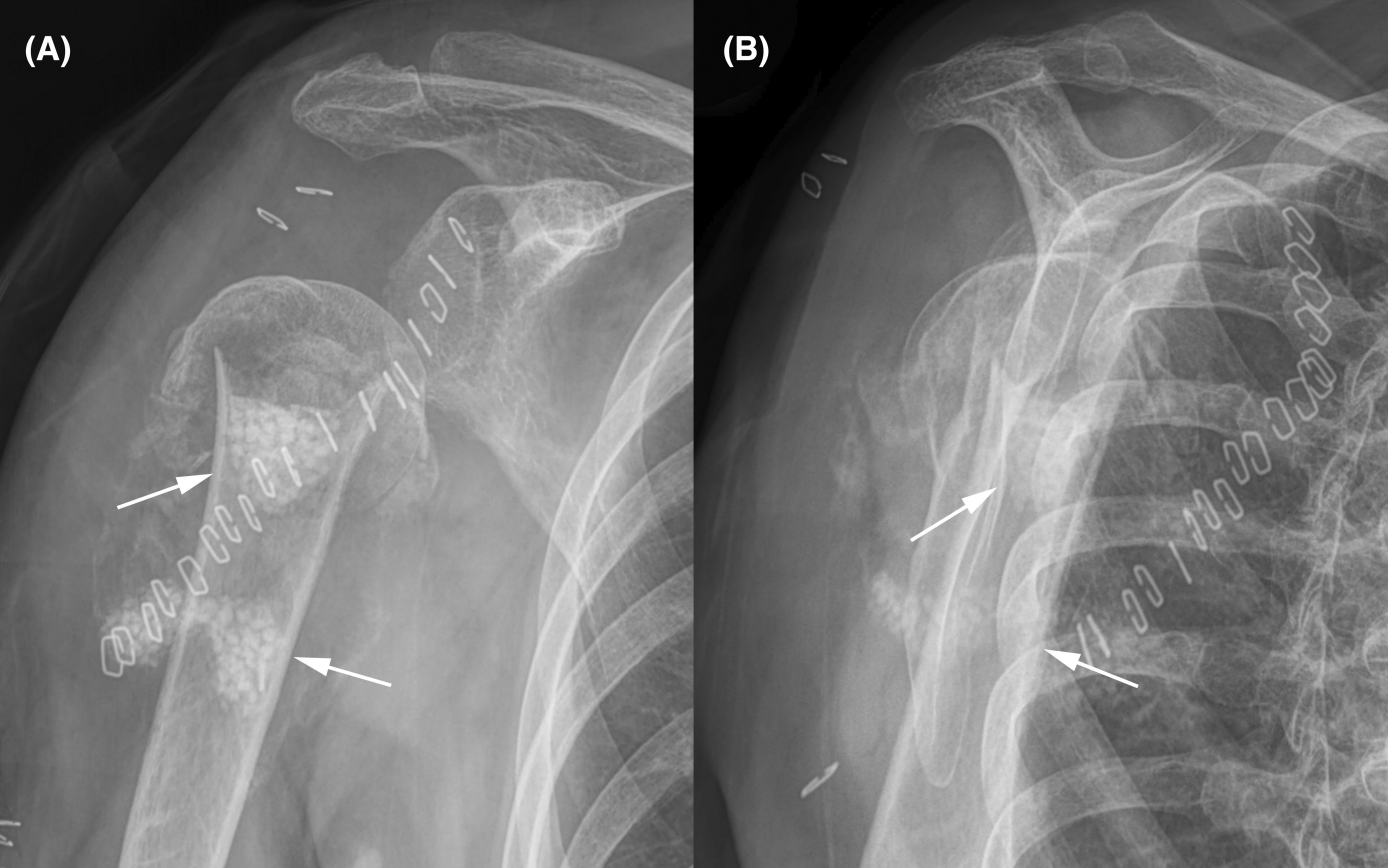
Anterior–posterior (A) and lateral (B) radiographs obtained at 2 weeks after the final debridement surgery (skin staples can be seen). The intramedullary calcium sulfate beads are indicated by the arrows. Note some of the beads subsided into the proximal diaphyseal medullary canal.
